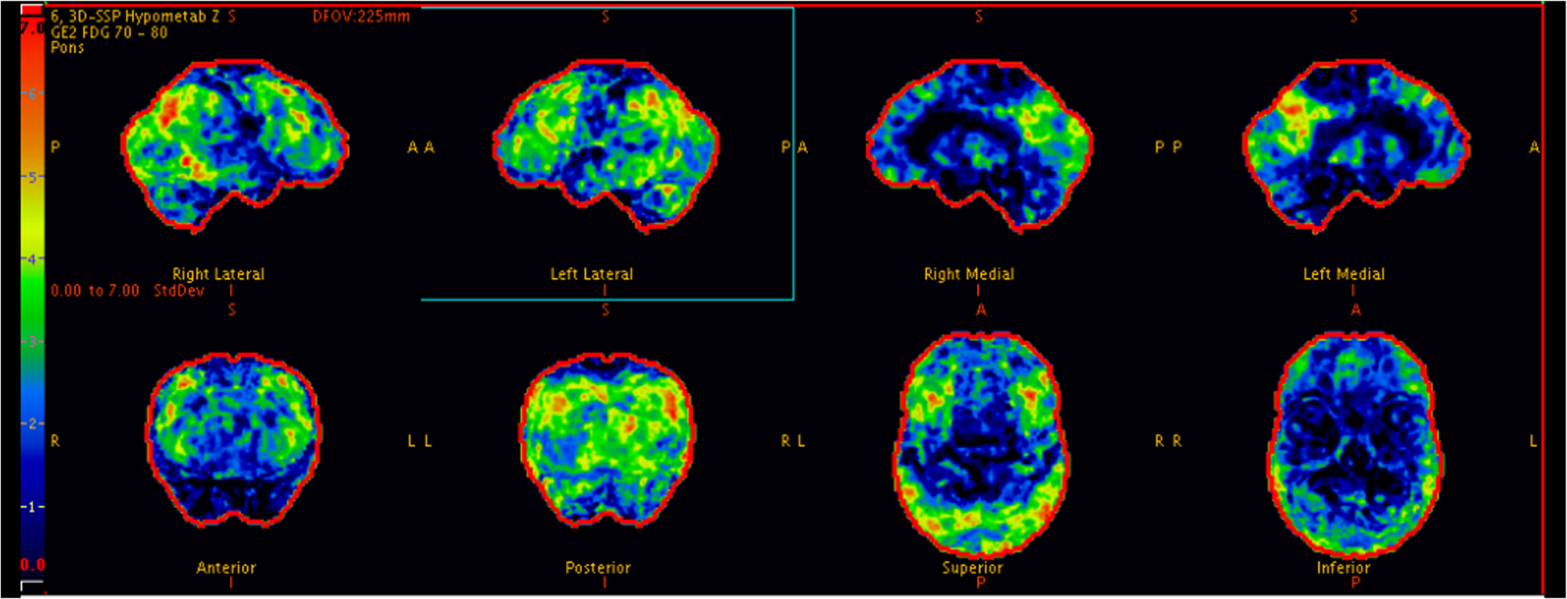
3D Stereotactic Surface Projection of FDG PET of our patient showed hypometabolism in bilateral occipital lobes, posterior parietal lobes, and to a lesser extent, bilateral frontal lobes. The metabolism in posterior cingulate gyri and bilateral temporal lobes is preserved. These findings were supportive of Dementia with Lewy Body (DLB). Involvement of the frontal lobes goes against this being a Posterior Cortical Atrophy variant of Alzheimer’s dementia
